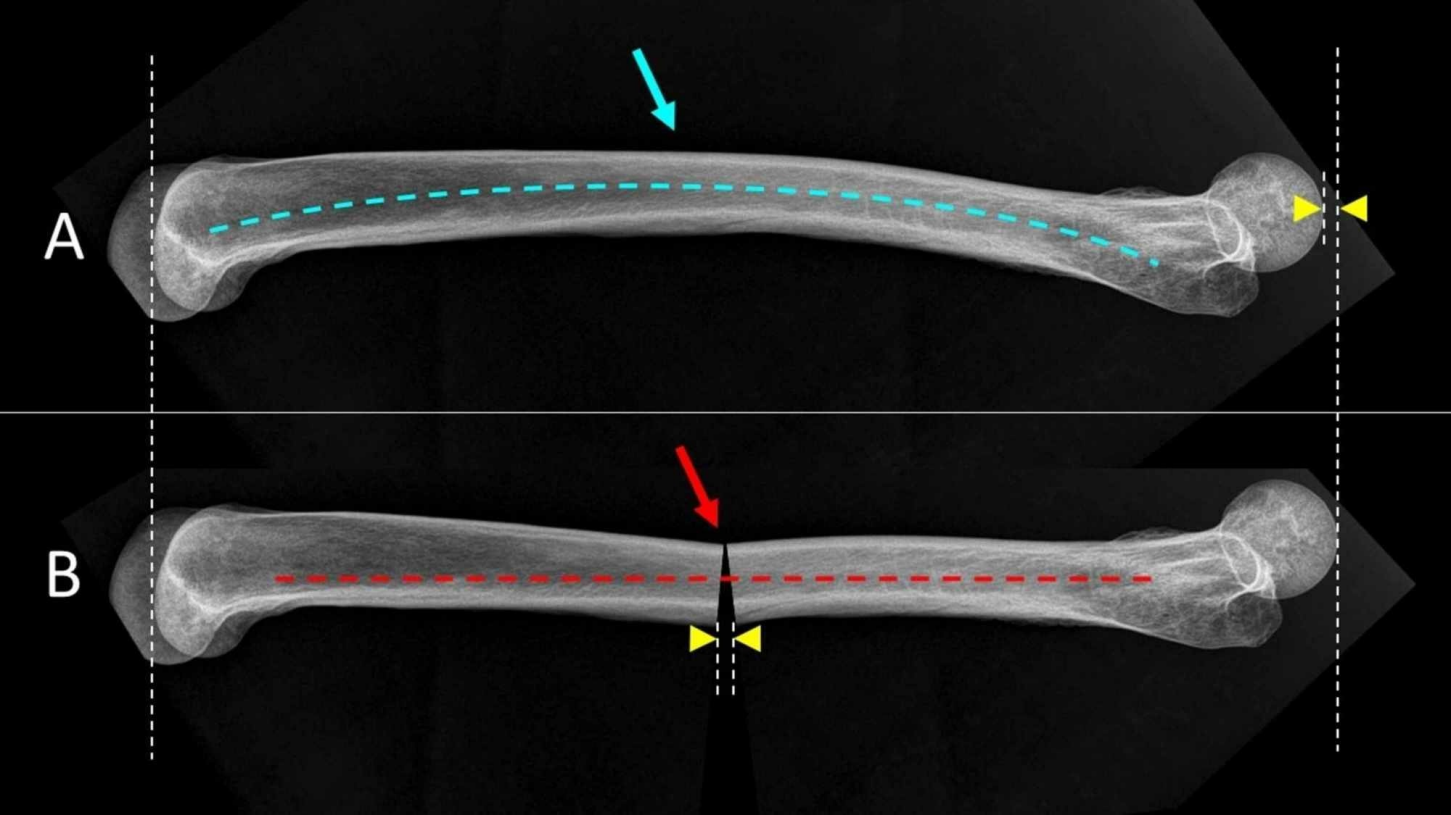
The effect of femoral antecurvatum on the length of the femur is shown on the lateral X-ray of a femoral bone A. Normal femoral antecurvatum. B. Loss of femoral antecurvatum after ESIN A. Blue arrow indicates normal femoral antecurvatum (blue dashed line), B. Red arrow indicates virtual transverse midshaft femoral fracture. Osteosynthesis with ESIN (red dashed line) leads to loss of the anterior curvature of the femur, either because the titanium nails are straight on the sagittal plane or secondary to the inherent sagittal instability of the bone-nail construct when treated with the ESIN technique. As a result, the femur becomes relatively longer than prior to the fracture (arrowheads). ESIN: elastic stable intramedullary nailing.
